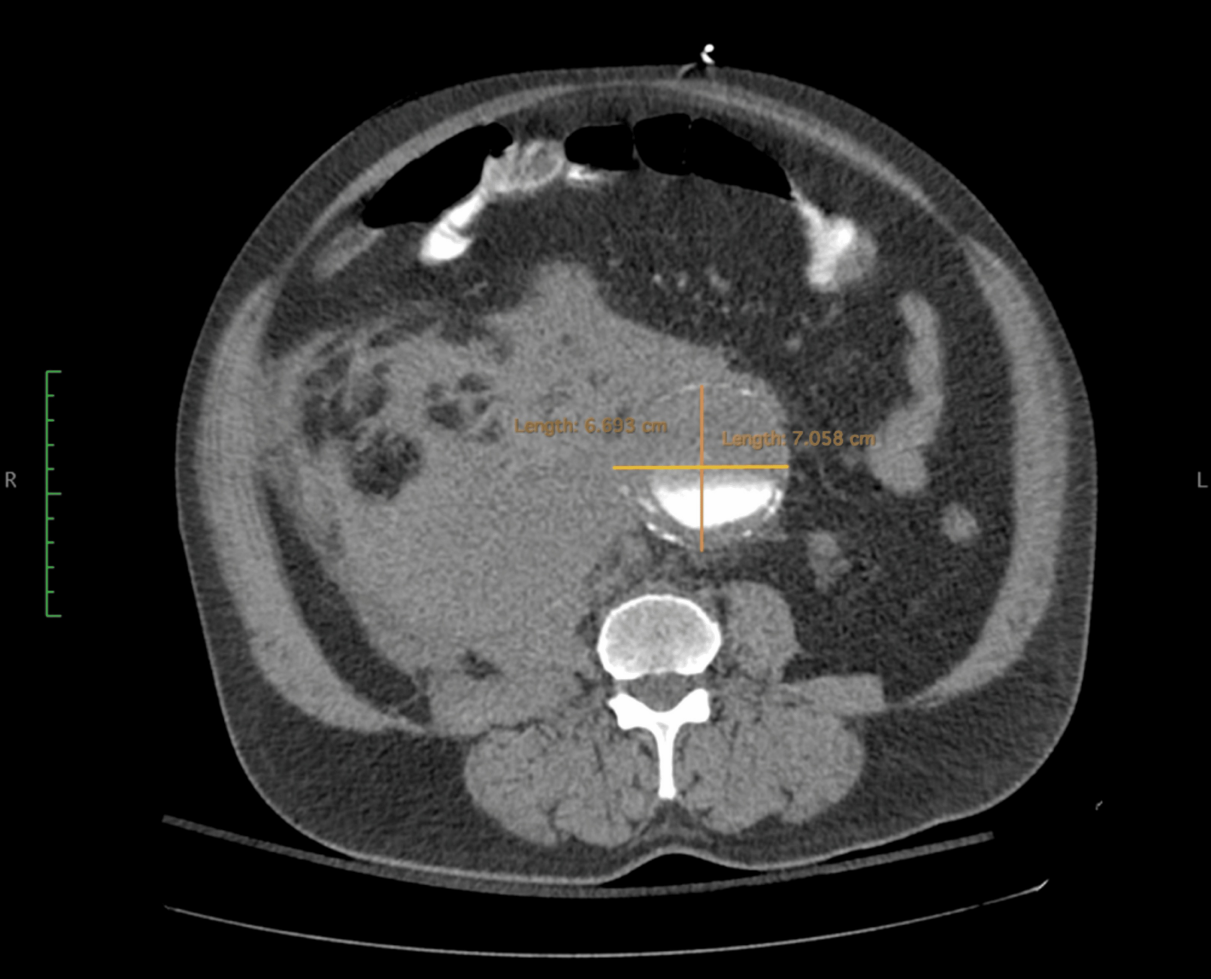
Computed tomography angiography showing an infrarenal abdominal aortic aneurysm measuring 70 mm in maximum diameter, with a contained rupture and associated retroperitoneal hematoma (estimated volume: 760 mL)
